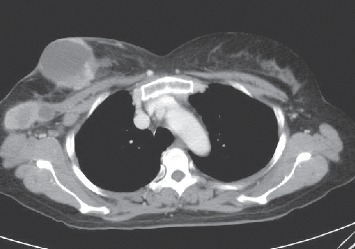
CT scan showed mixed solid-cystic mass in the right breast with matted necrotic right axillary node involvement.
